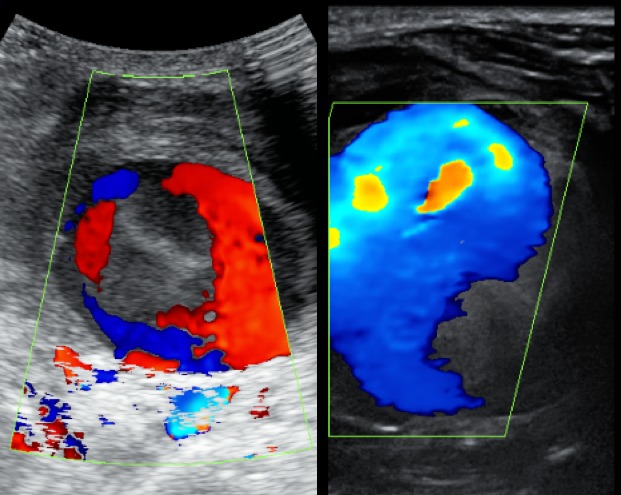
Turbulence au doppler couleur réalisant un aspect de Ying-Yang
